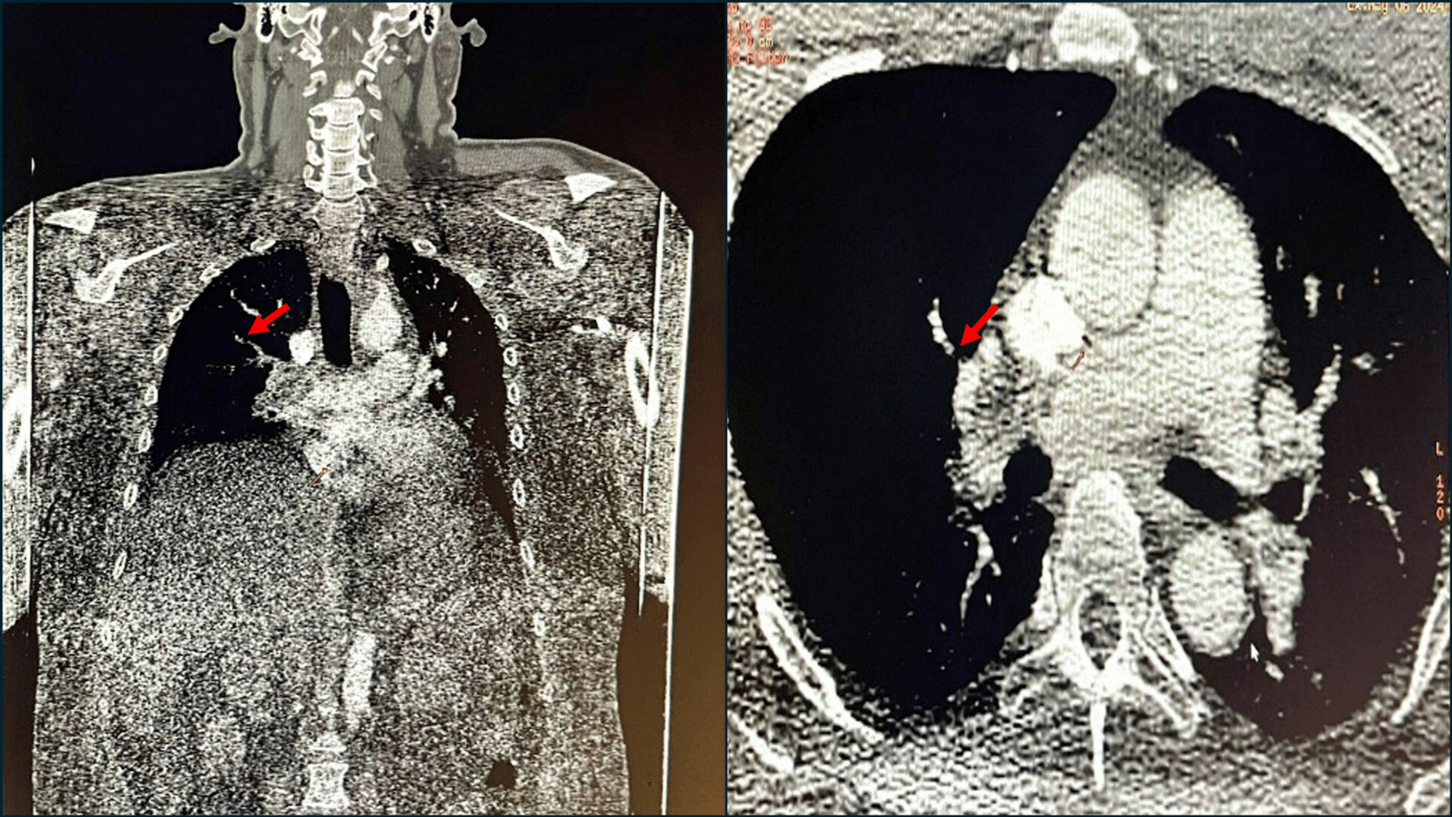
Thoracic CT angiography. (A) Coronal and (B) axial sections demonstrating bilateral pulmonary thromboembolism, with obstruction of both main pulmonary arteries (red arrows).
